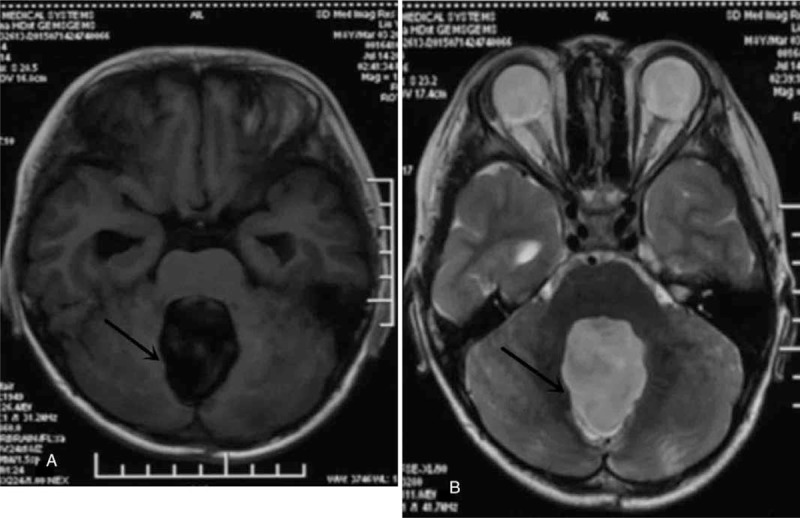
Postoperation MRI A. T1-weighted sequences B. T2-weighted sequences. MRI = magnetic resonance imaging.
